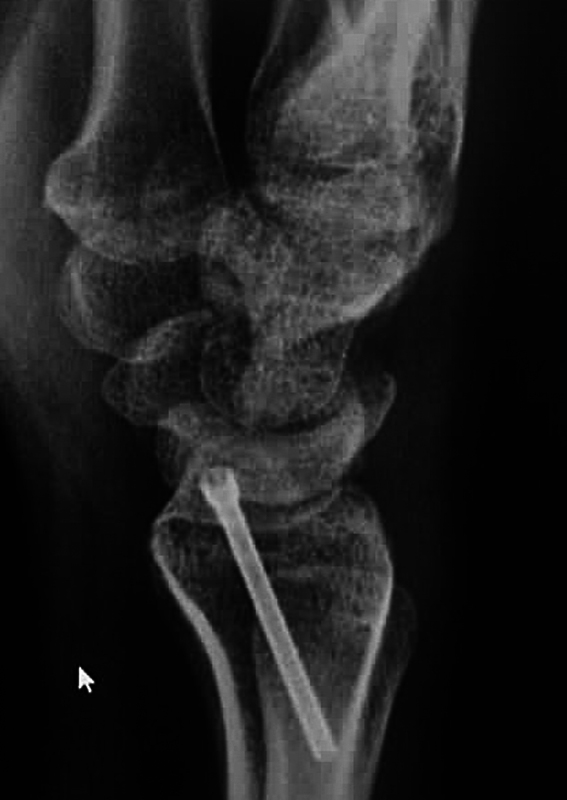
Postoperative aspects of wrist radiography in lateral view.
